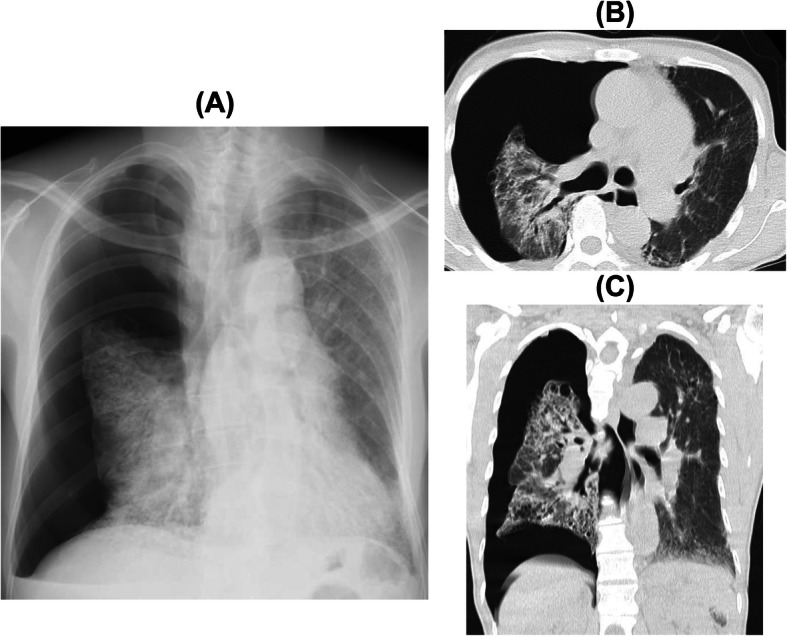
a Chest X-ray of the patient upon the development of pneumothorax. b Axial and c coronal views of chest computed tomography at the development of pneumothorax
